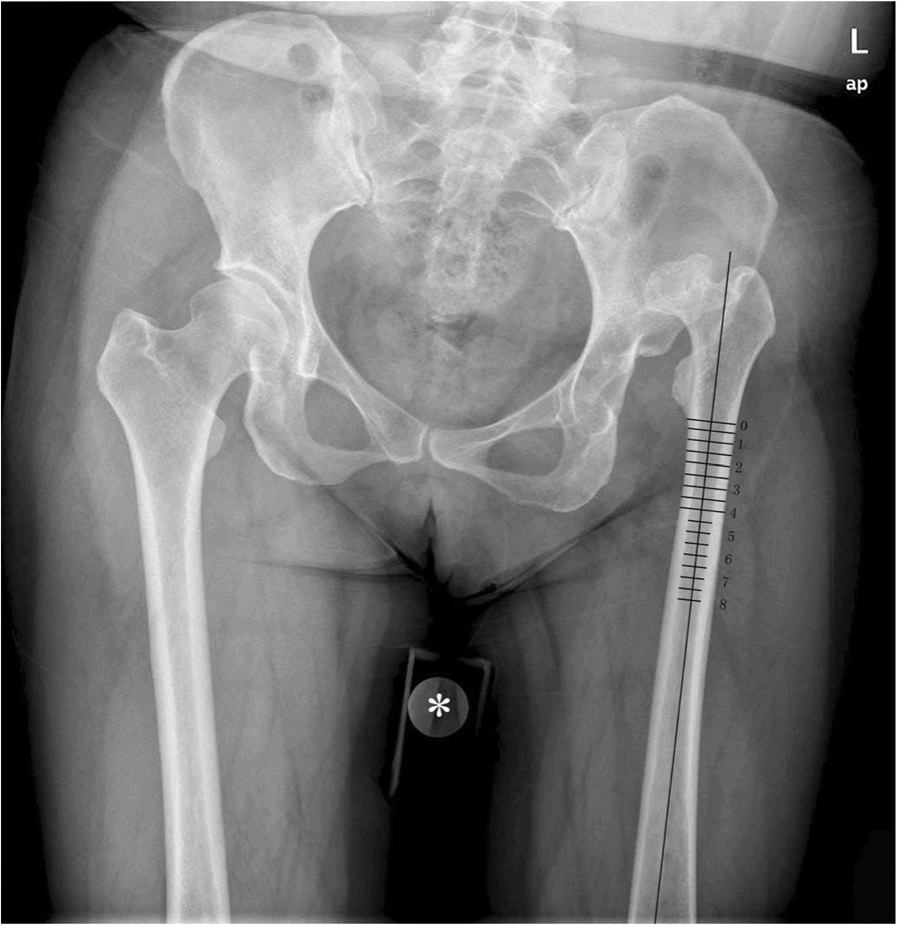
The inner and external diameters of each level of osteotomy on anteroposterior view on the radiograph. * A fixed reference, one-yuan coin
